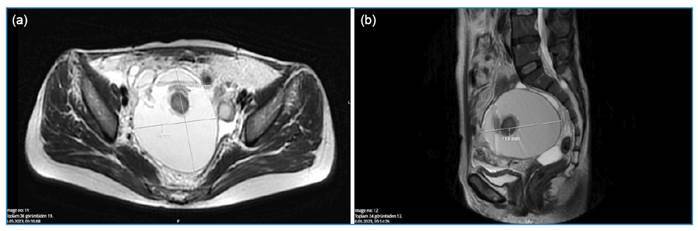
Mature ovarian cystic teratoma on T2-weighted axial (a) and sagittal (b) magnetic resonance imaging.
